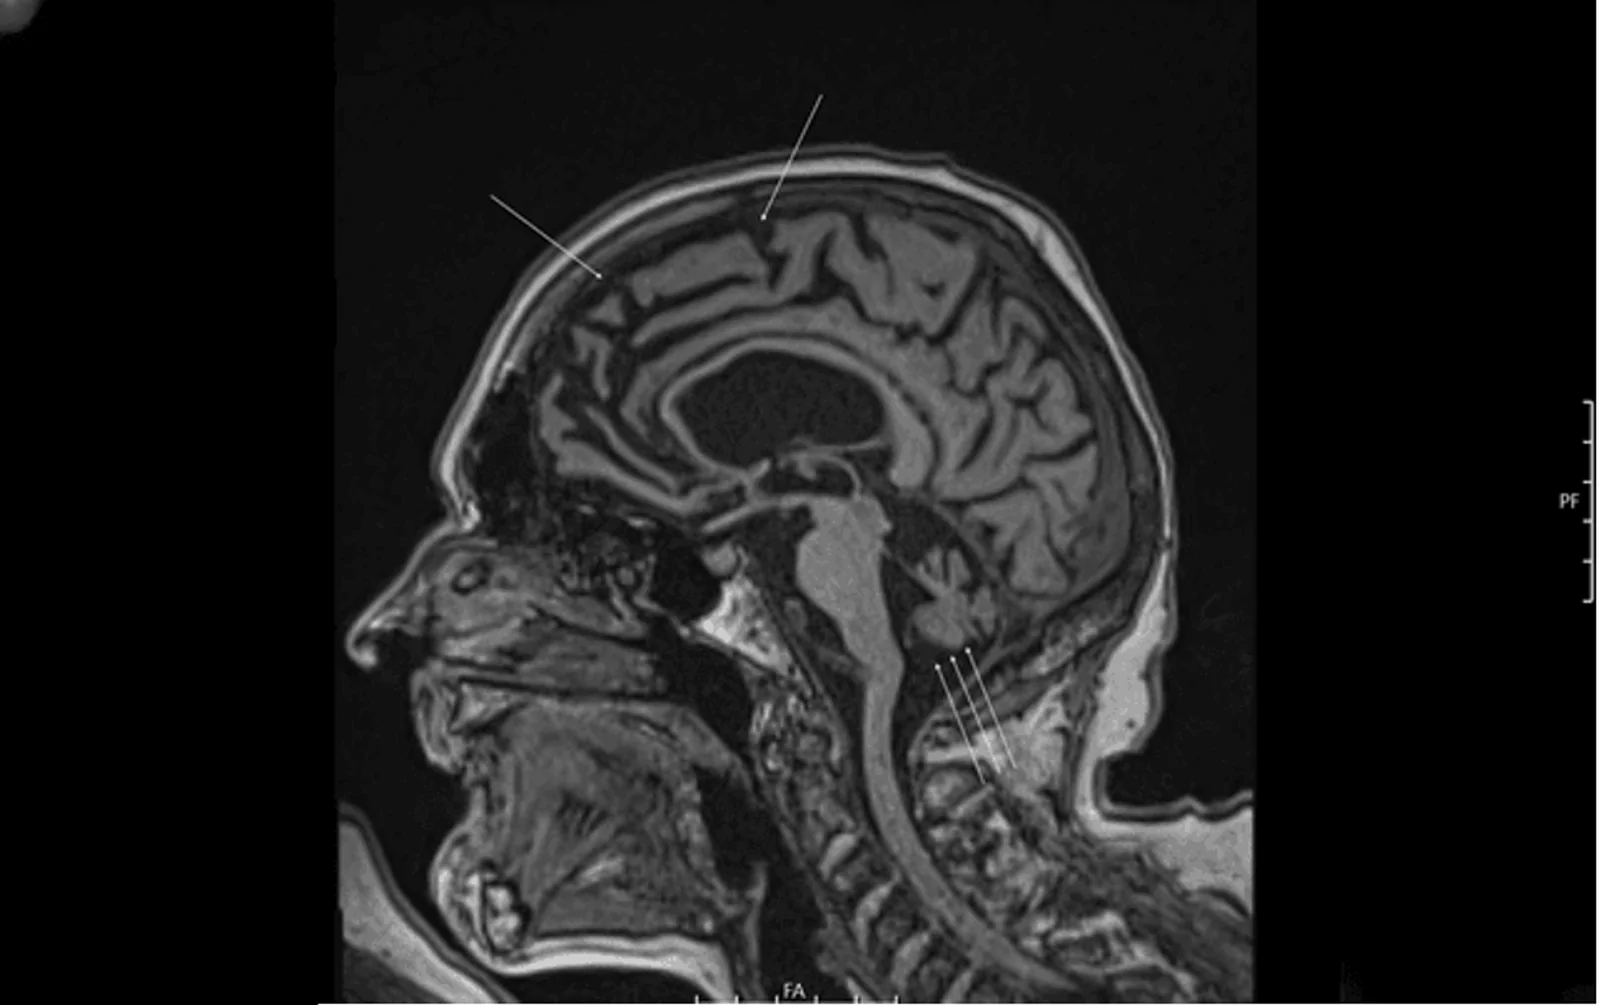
MRI of the head performed at the age of 76. White arrows show generalized brain volume loss with striking frontotemporal atrophy and diffuse cerebellar atrophy.
